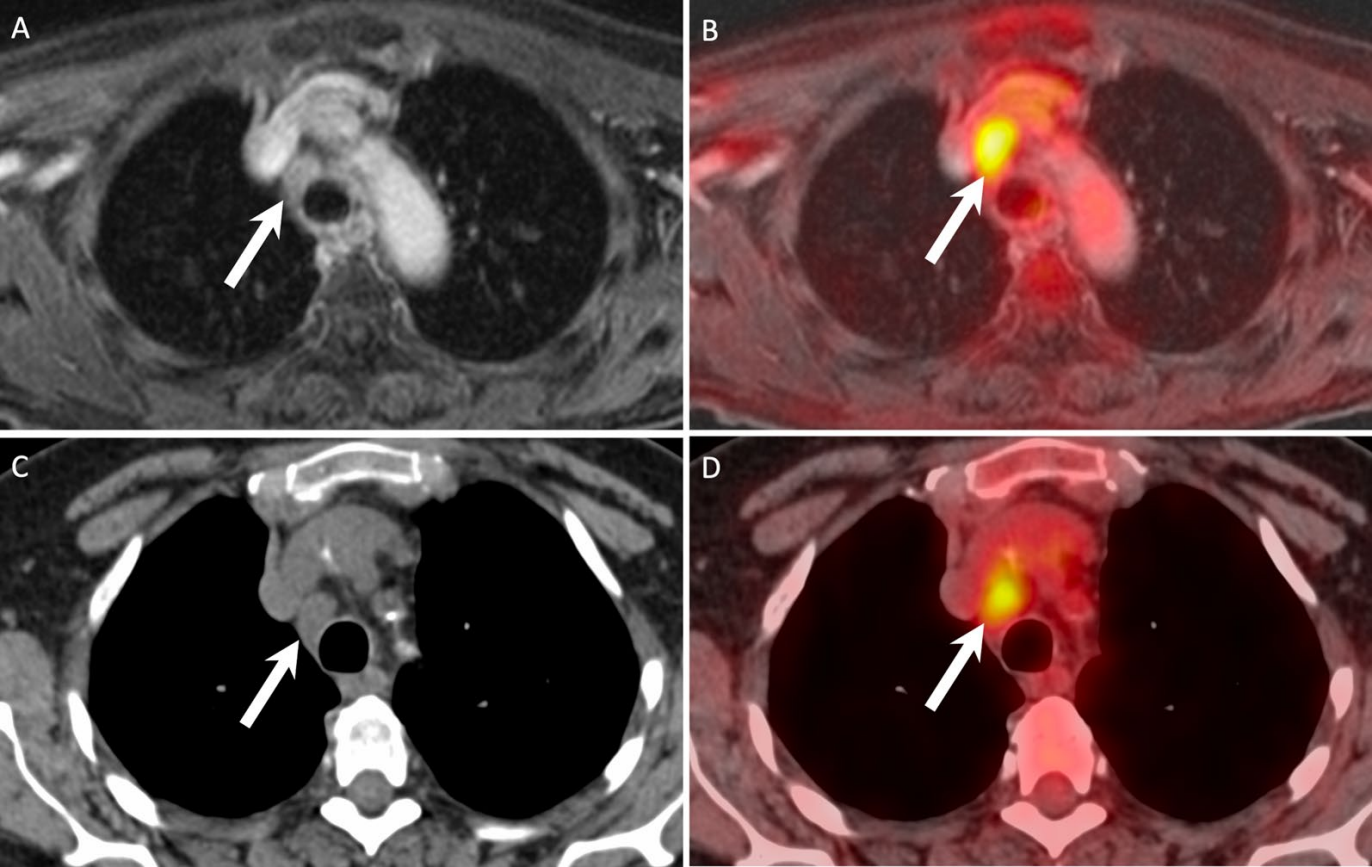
False positive PET/MRI (A, B) and PET/CT (C, D) in a 63-year-old female imaged for follow-up of a SCC of the larynx (T3N1) treated with radiochemotherapy. Both PET/MRI and PET/CT were rated as positive for distant mediastinal lymph node metastases. An enlarged mediastinal lymph node (arrows) shows a combination of high focal [^18^F]FDG uptake (SUVmax on PET/MRI = 7.5 and SUVmax on PET/CT = 6.7) and slightly heterogeneous contrast enhancement on the contrast-enhanced fat-saturated MR image. On the corresponding CT image, due to the absence of contrast enhancement, only lymph node enlargement was present (13 × 15 × 17 mm). The lymph node was rated with a score of 5 on PET/MRI and a score of 4 on PET/CT. However, mediastinoscopy with biopsy revealed sarcoidosis
